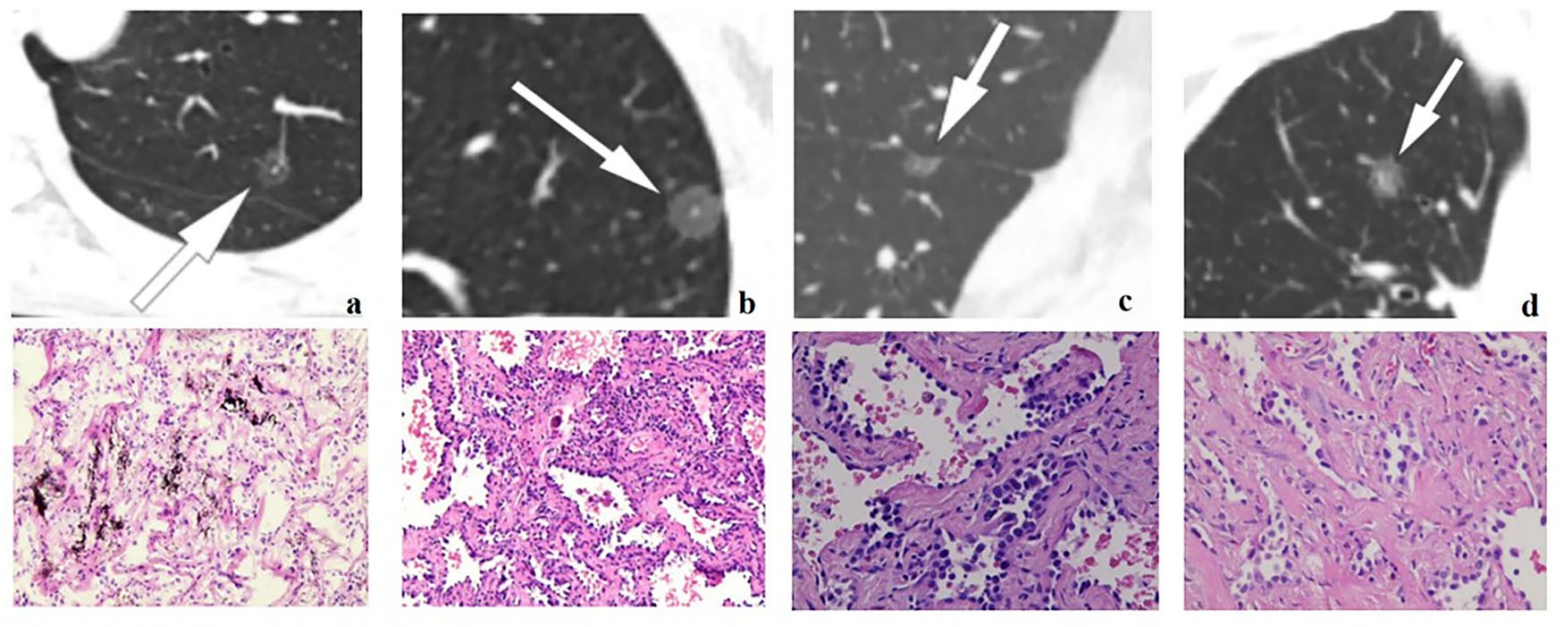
The CT images of AAH, AIS, MIA and IAC and their corresponding pathological sections. a–d The CT images of AAH, AIS, MIA, and IAC, respectively, and the corresponding lower images show the pathological images. As can be seen from the above figure, pure ground-glass nodules that appear similar on CT images have different pathological findings (the high-density area within the nodule in the above figure was confirmed to be vascular track signs rather than a solid component on serial tomography images), which is difficult to identify by visual assessment by the radiologist alone
